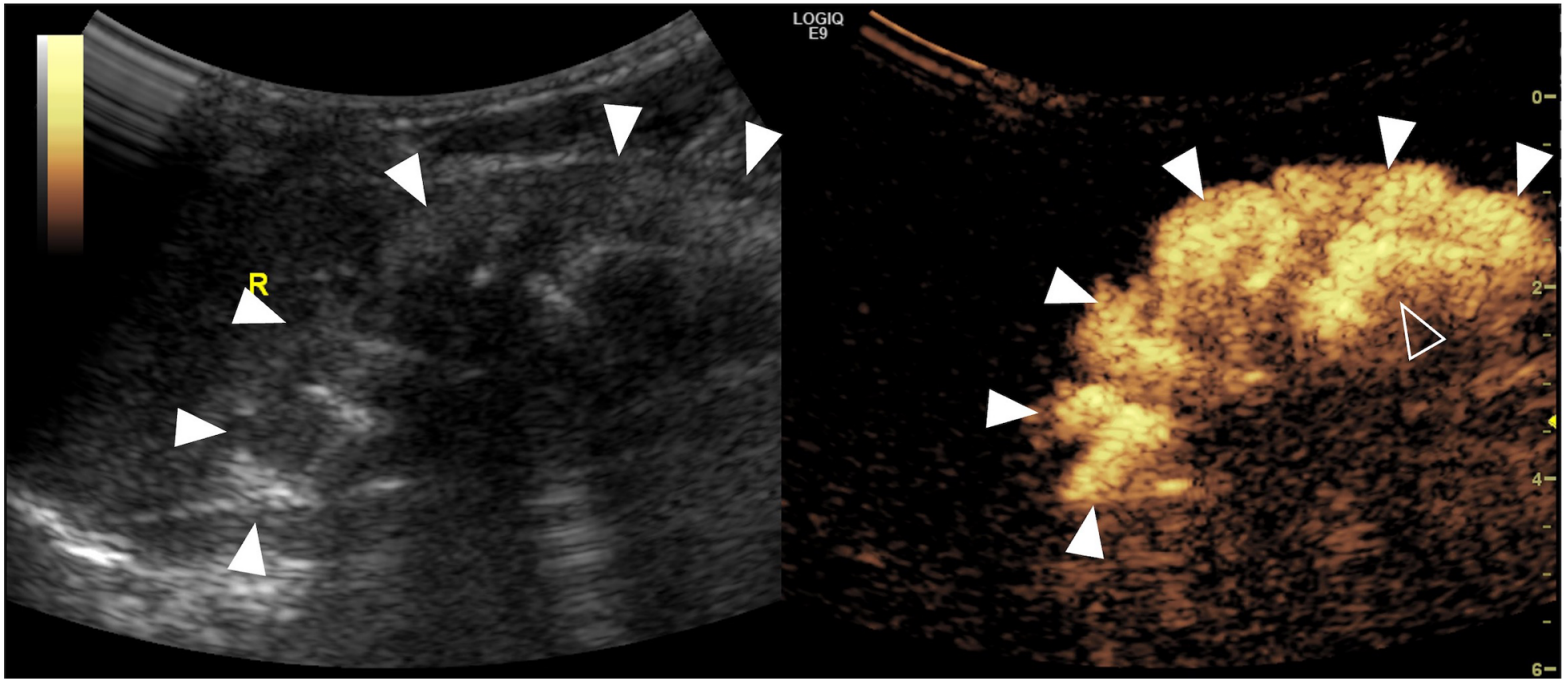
Severe intrarenal reflux complicated with vesicoureteral reflux on contrast-enhanced voiding urosonography of the dual-screen mode of the right kidney in a 14-day-old girl. Sagittal images of the right kidney show microbubbles refluxing into the pelvicalyceal system (open arrowheads) and diffusing into the entire renal parenchyma (solid arrowheads).
